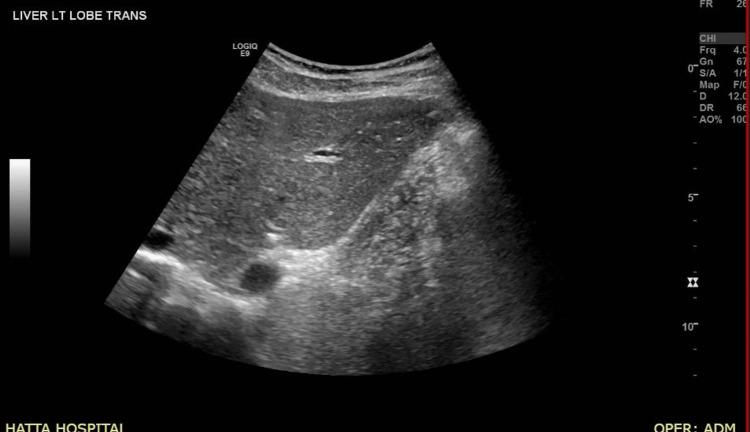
Ultrasonographic Image of the Liver (Left Lobe - Transverse View) The image reveals hepatomegaly, characterized by an enlarged liver with a mildly heterogeneous echotexture.
